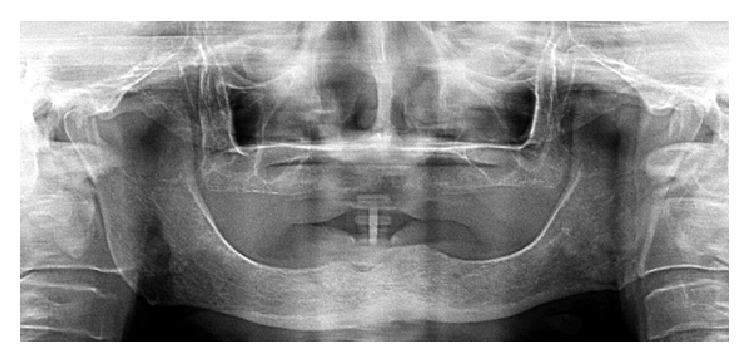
Panoramic radiography revealing bone neoformation after 6 months of the surgical procedure.
